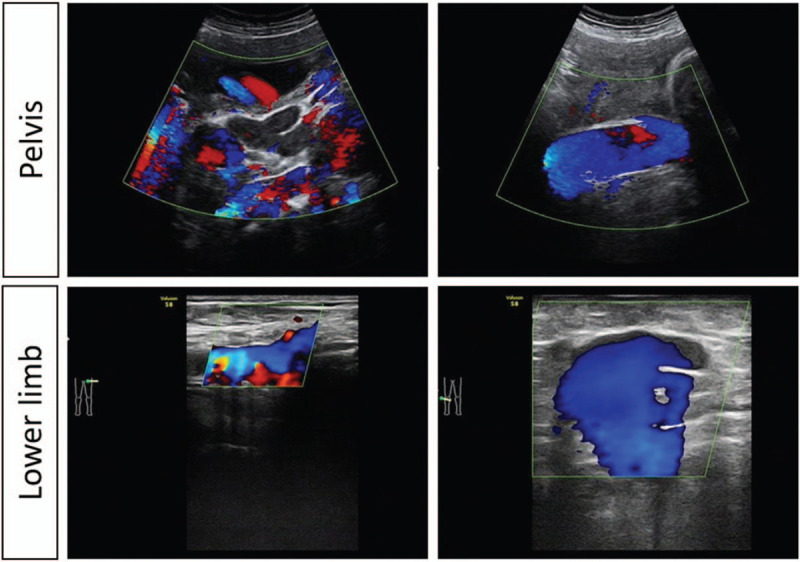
Ultrasonic view at 28 weeks of gestation.
